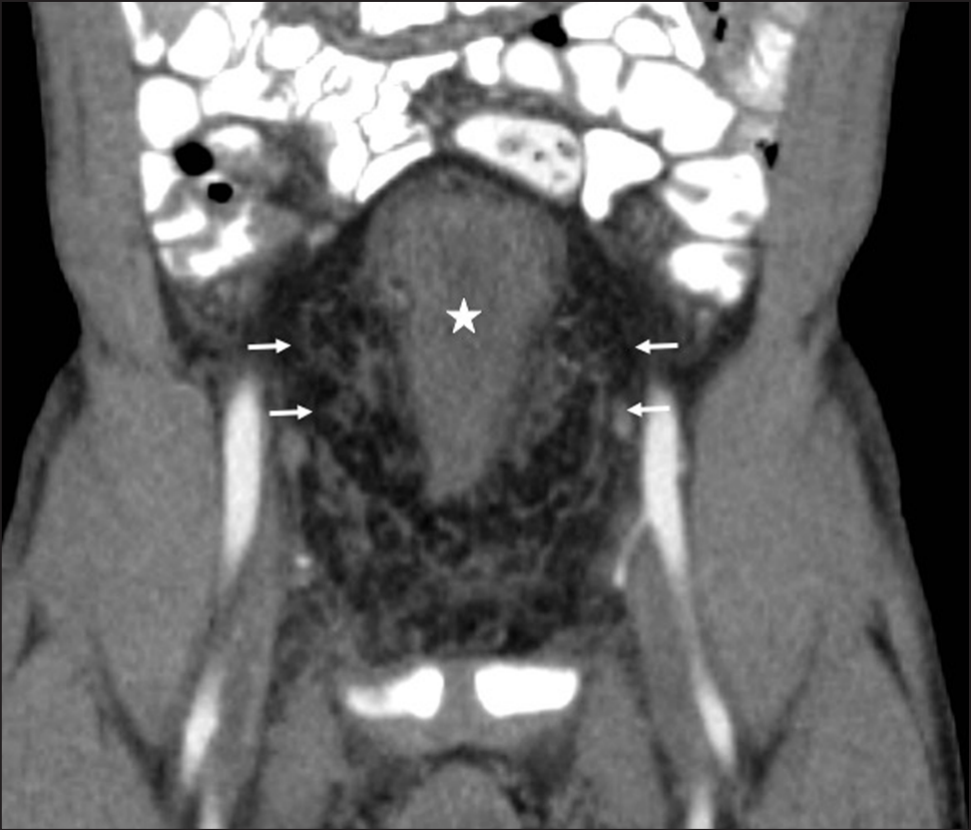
Contrast-enhanced computed tomography. Coronal imaging showing a ‘pear’-shaped bladder (white star) surrounded by hypodense fatty tissue (arrows).
